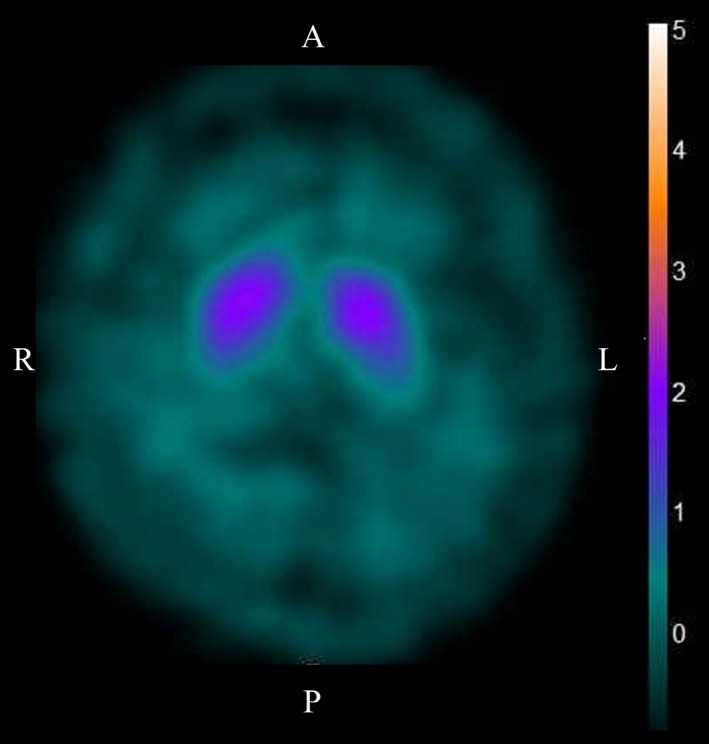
Reduced ^123^I‐ioflupane binding in the bilateral striata of Patient 3. Dopamine transporter SPECT with ^123^I‐ioflupane was performed in Patient 3. ^123^I‐ioflupane binding was reduced in the bilateral striata, with left‐sided predominance. The specific binding ratio was 3.68 on the right and 2.66 on the left side; the asymmetry index was 32.2%
